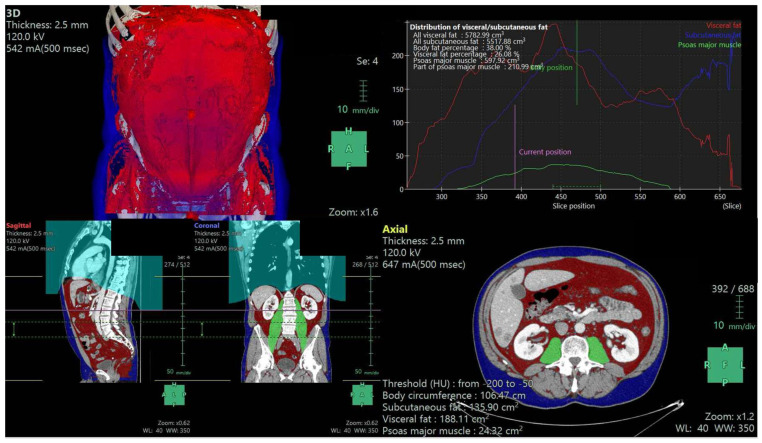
User interface in the extraction of 3D BCPs. The graphic shows the 3D (upper left panel) and 2D multiplanar reconstruction (lower panels) models of the semi-automated BCp extraction from a CT scan of the abdomen. Upper right panel shows the volume distribution of each major compartment (visceral fat, subcutaneous fat, and psoas muscle) throughout the z-axis. Note how variable the ratio between visceral and subcutaneous fat areas is, depending on what level is chosen to perform the measurement.
